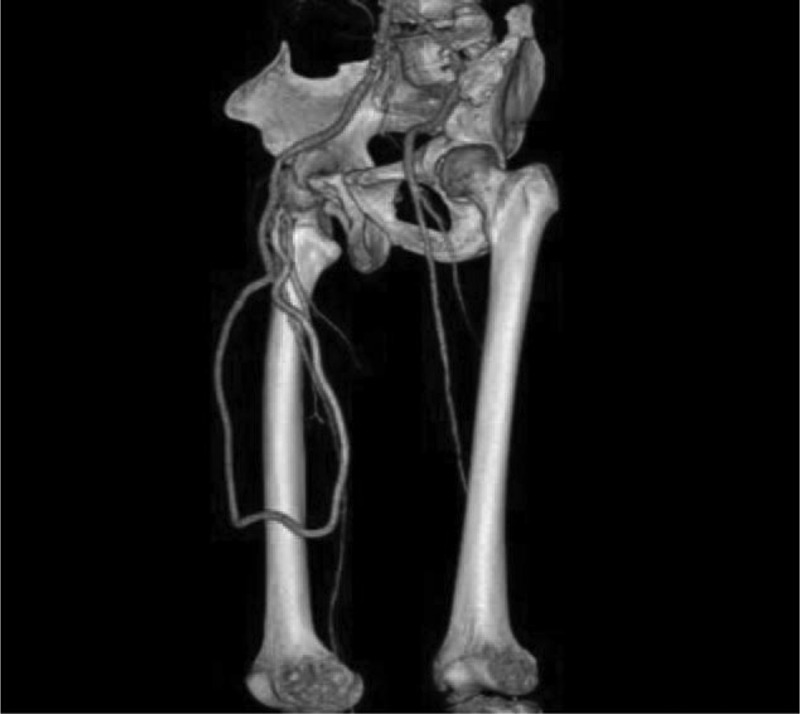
Computed tomography angiography (CTA) of the femoral artery-deep femoral artery arterioarterial prosthetic loop 1 year after placement.
